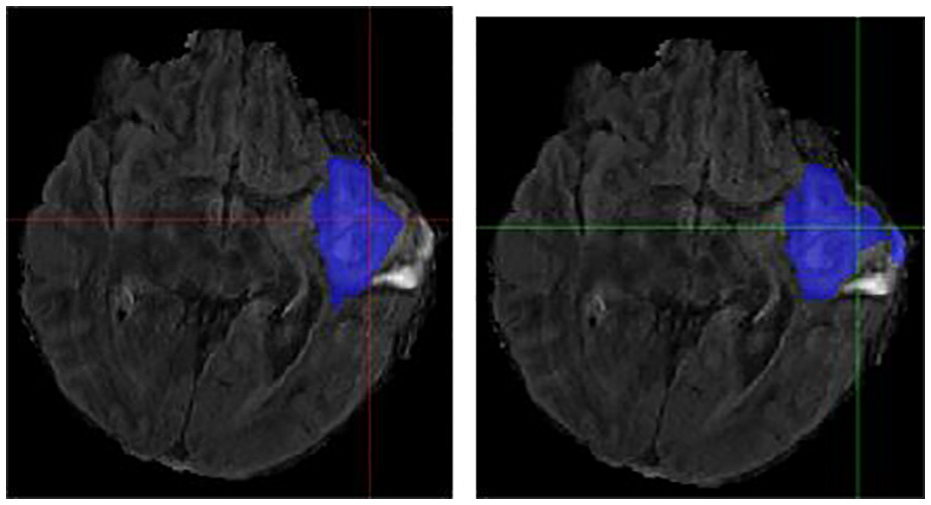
Case One (Worsened). Flair MRI. rMR only (Left) and Total SynMR MRI (Right) DSC of WT changed from 0.76 to 0.58.
